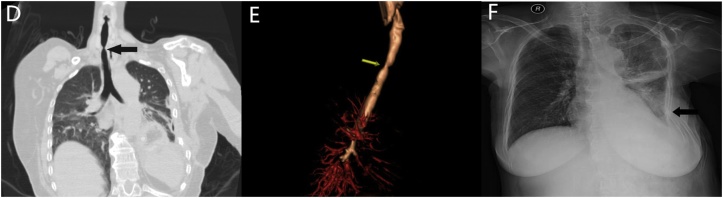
(D) and (E) CT showing the occurrence of tracheostenosis; (F) X-ray showing chest collapse after thoracoplasty and a normal tracheal morphology.
